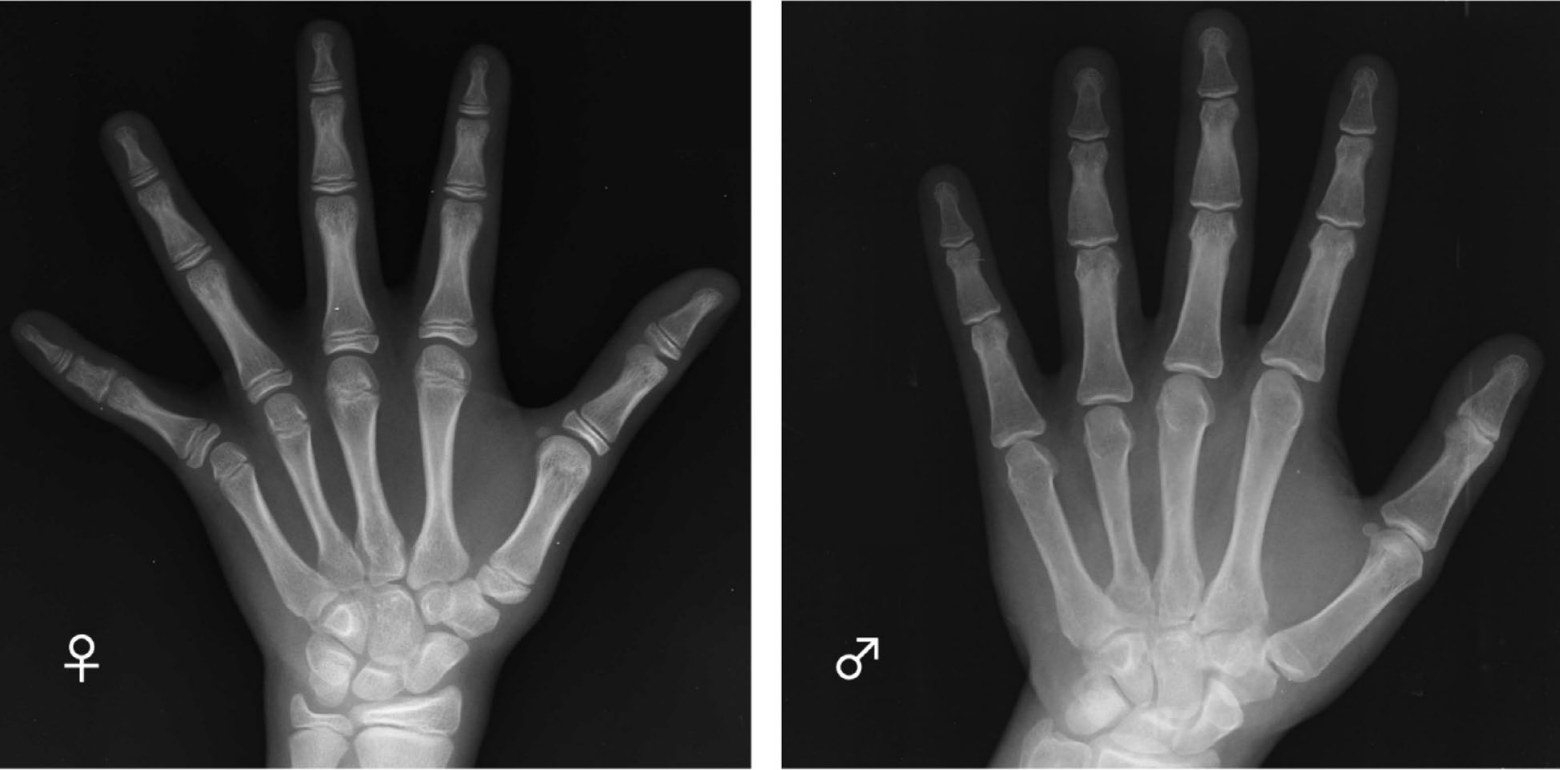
Example hand X-rays in the Georgian Bone Age Dataset. Left: A girl with chronological age of 117 months, reference bone age of 138 months, and *Deeplasia-GE* bone age of 137 months. Right: A boy with chronological age of 213 months, reference bone age of 214 months, and *Deeplasia-GE* bone age of 216 months.
